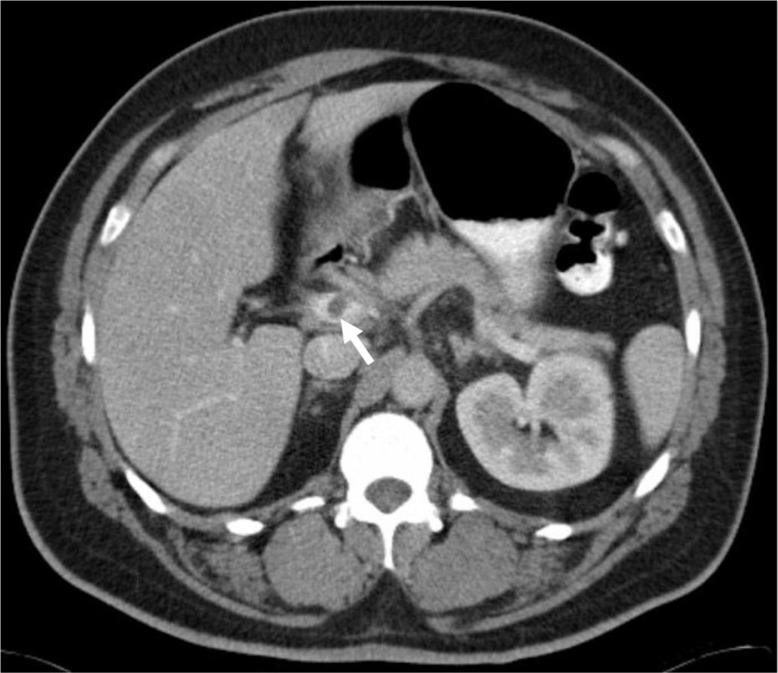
Contrast enhanced CT of the abdomen showing a nonocclusive thrombus within the main portal vein (white arrow).
